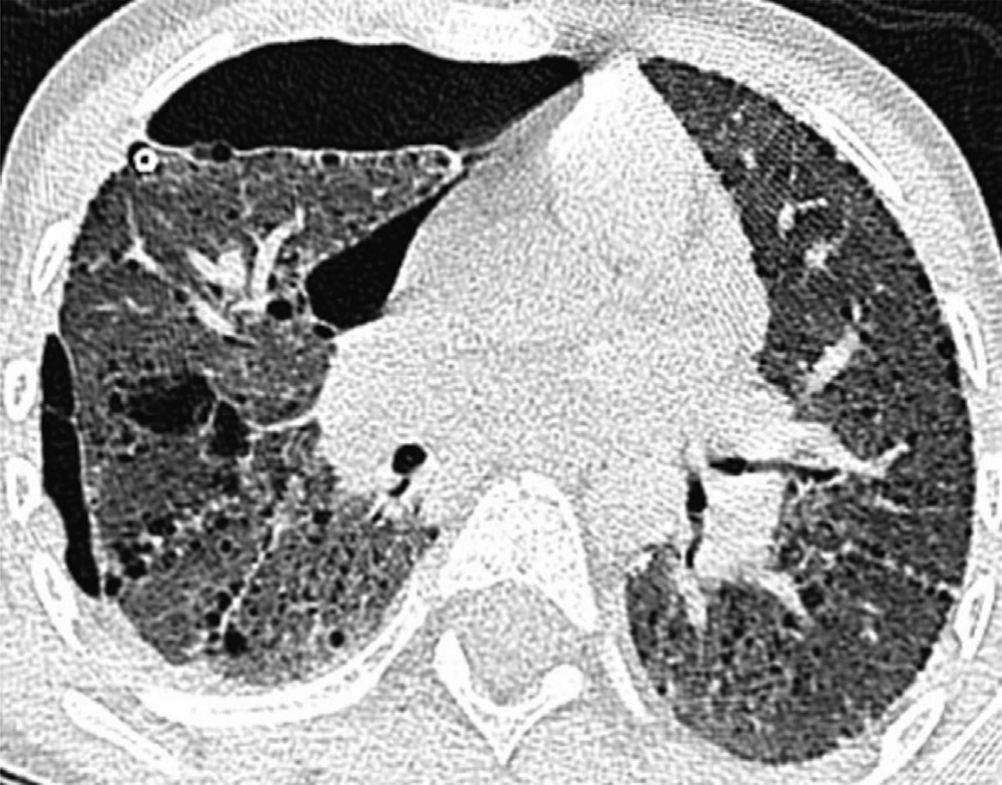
At 7 years of age (case 1), an axial noncontrast chest CT slice shows multiple thin-walled cysts of varying sizes, predominantly distributed in subpleural, juxtafissural, and peribronchovascular regions of both lungs, as well as a right-sided pneumothorax.
